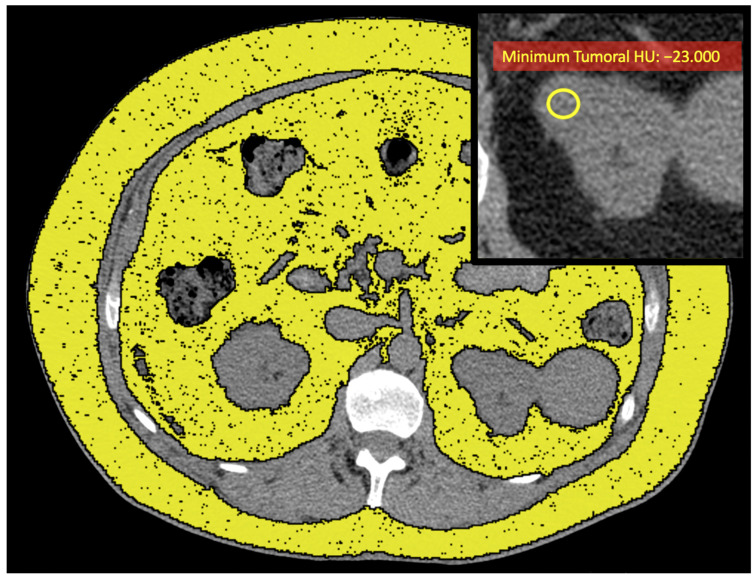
Unenhanced axial CT images of a patient with low-grade ccRCC with ADFP expression (same patient as in Figure 2A) show yellow ROIs highlighting the abdominal adipose tissue compartments, and the enlarged detail in the upper right shows the yellow ROI with a low minimum tumoral HU value (HU: −23).
